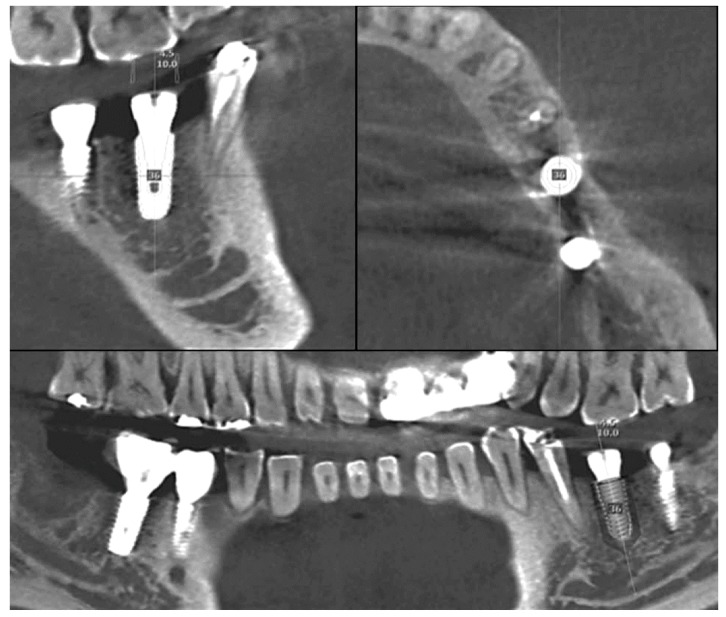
A library image of implant fixture is loaded on the post-operative CT. By this image replacing analysis, the implant placed position is determined.
